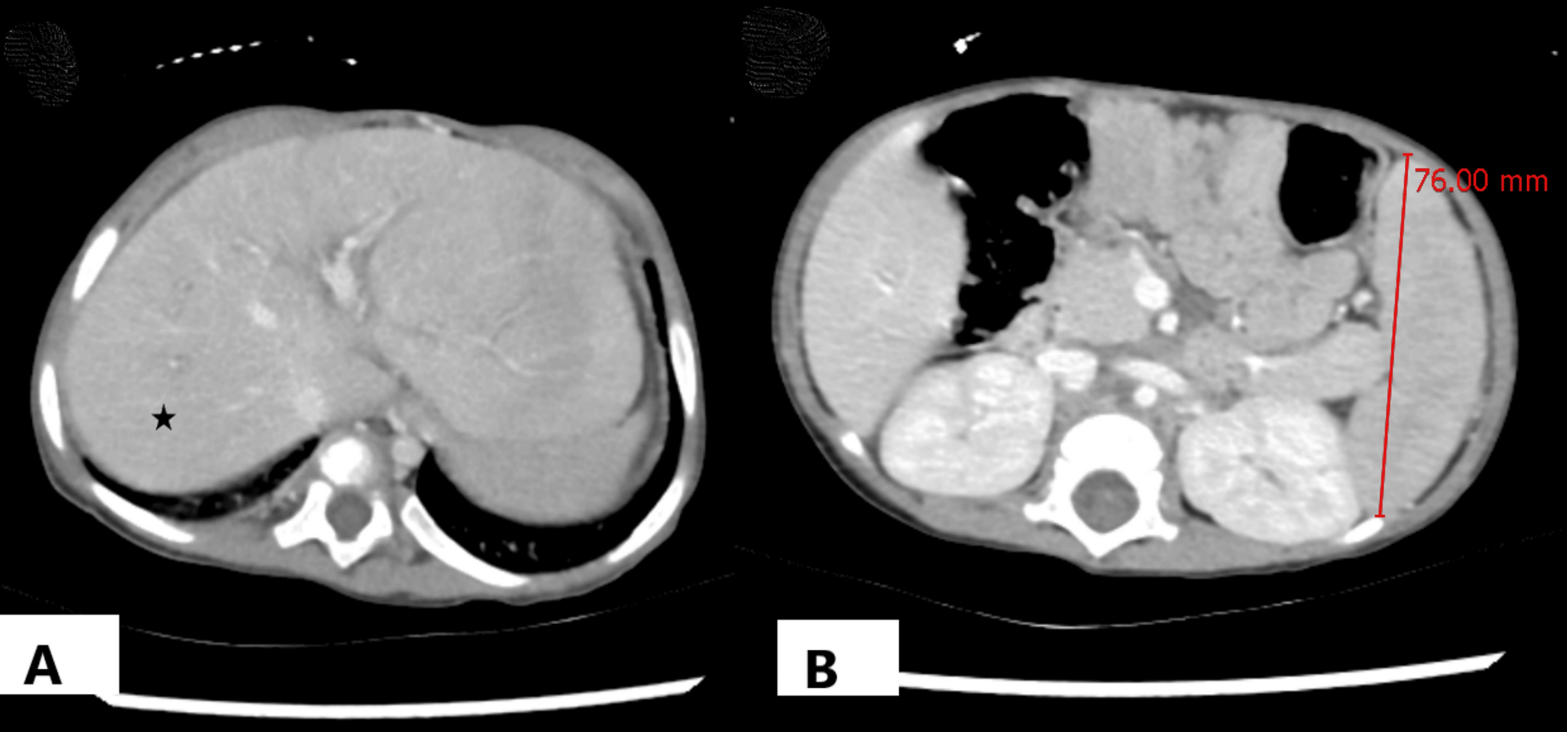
CT images of the abdomen (A) Slightly undulating hepatic margins without frank cirrhosis (asterisk shows normal liver parenchyma) associated with mild splenomegaly, (B) No focal hepatic lesion or intra-/extra- hepatic biliary dilatation was noted.
